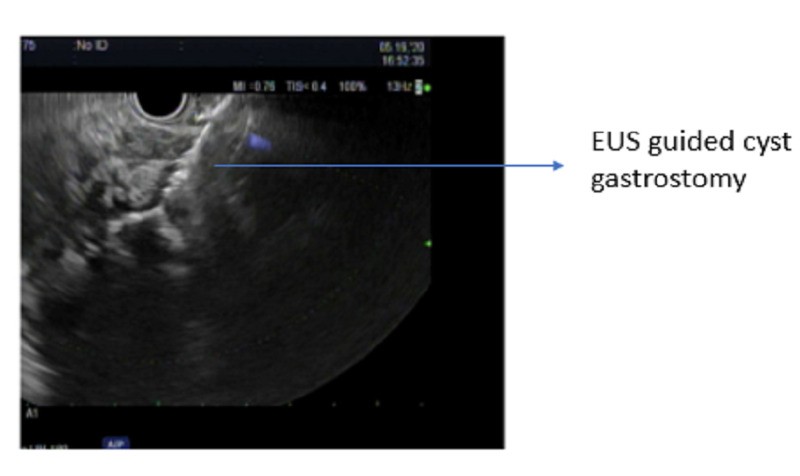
Endoscopic ultrasound guided cystogastrostomy.
